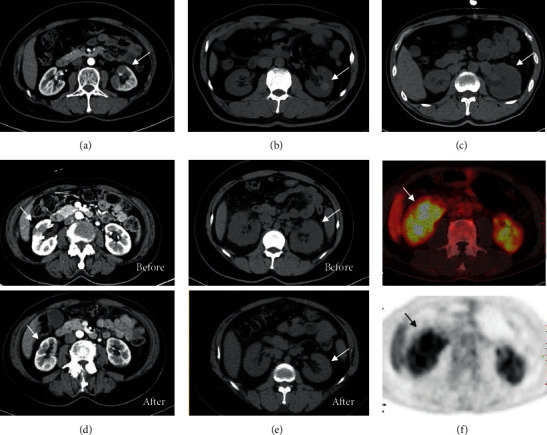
(a) Patchy low-density lesion in contrast-enhanced CT scan of IgG4-RKD (arrow). (b) Nodule lesion in renal parenchyma of IgG4-RKD (arrow). (c) Arrow is pointed at the thickening perirenal capsule of an IgG4-RKD patient. (d) Repeated contrast-enhanced CT scan revealed radiological remission of an IgG4-RKD patient after 1-year treatment. Arrow is pointed at the low-density lesion area before and after treatment. (e) Repeated CT scan of IgG4-RKD after 2 years. The swelling of the bilateral kidney prominently ameliorated. (f) PET-CT of an IgG4-RKD patient presenting with unilateral renal mass with high uptake of 19F-FDG (arrow pointed at the mass).
